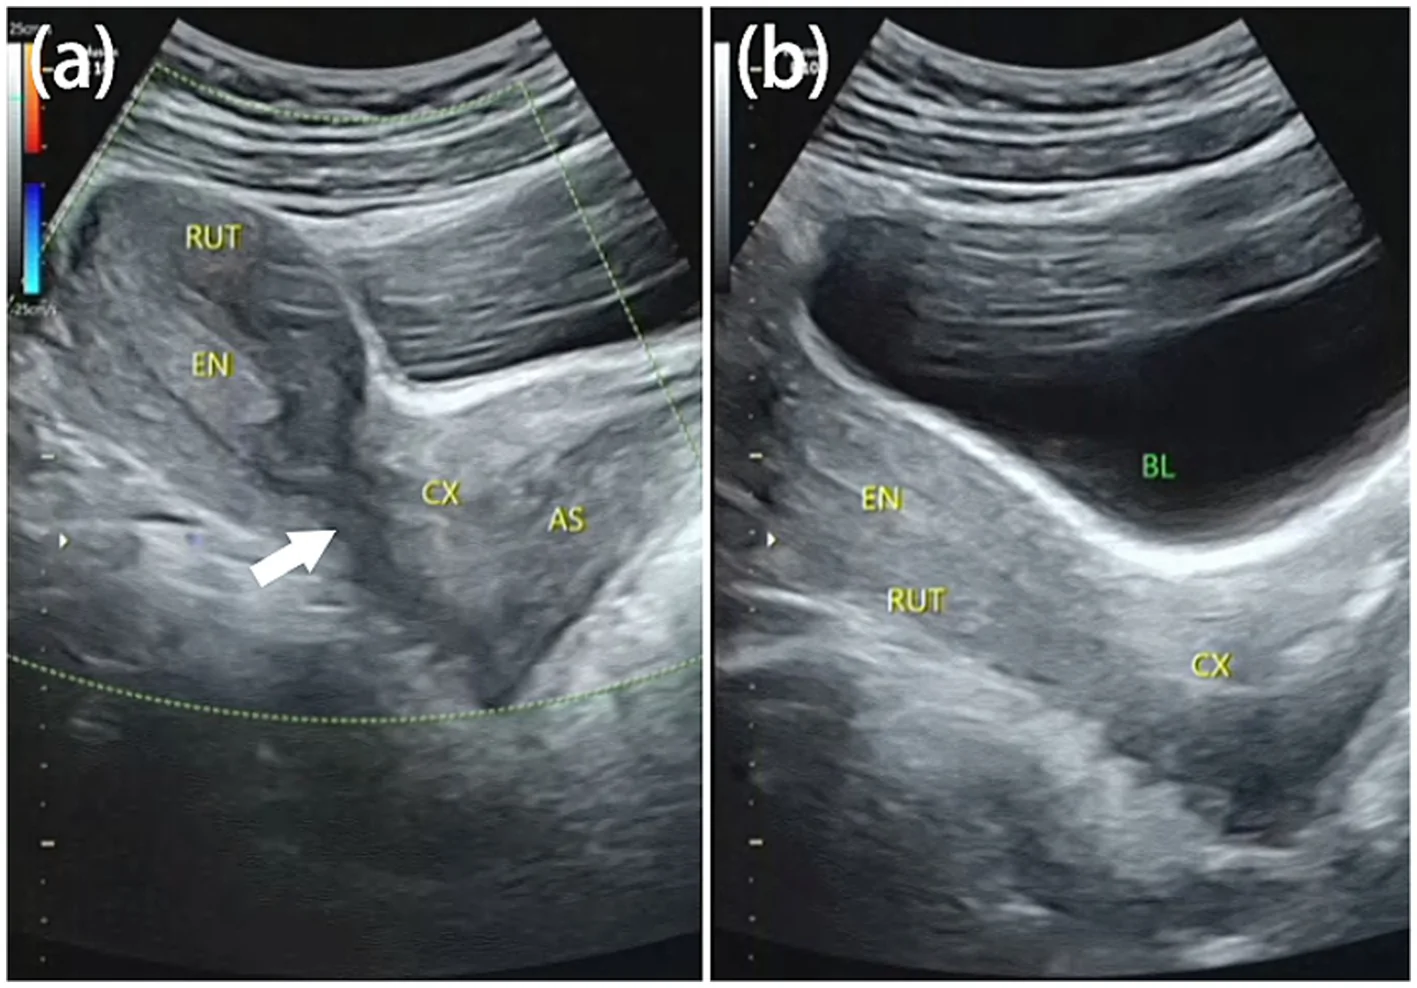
Ultrasound findings (a) Preoperative scan showed a fluid-filled dark area in the right vagina (hematocolpos, white arrow). (b) Post-menstruation scan (2 months) verified no residual hematocolpos or hematometra.
